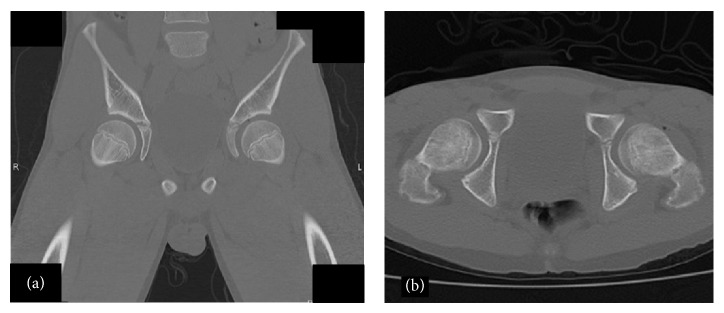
Coronal (a) and axial (b) CT scan after reduction shows the left femoral head correctly positioned in the acetabulum with no fractures or interposition of osteochondral fragments.
